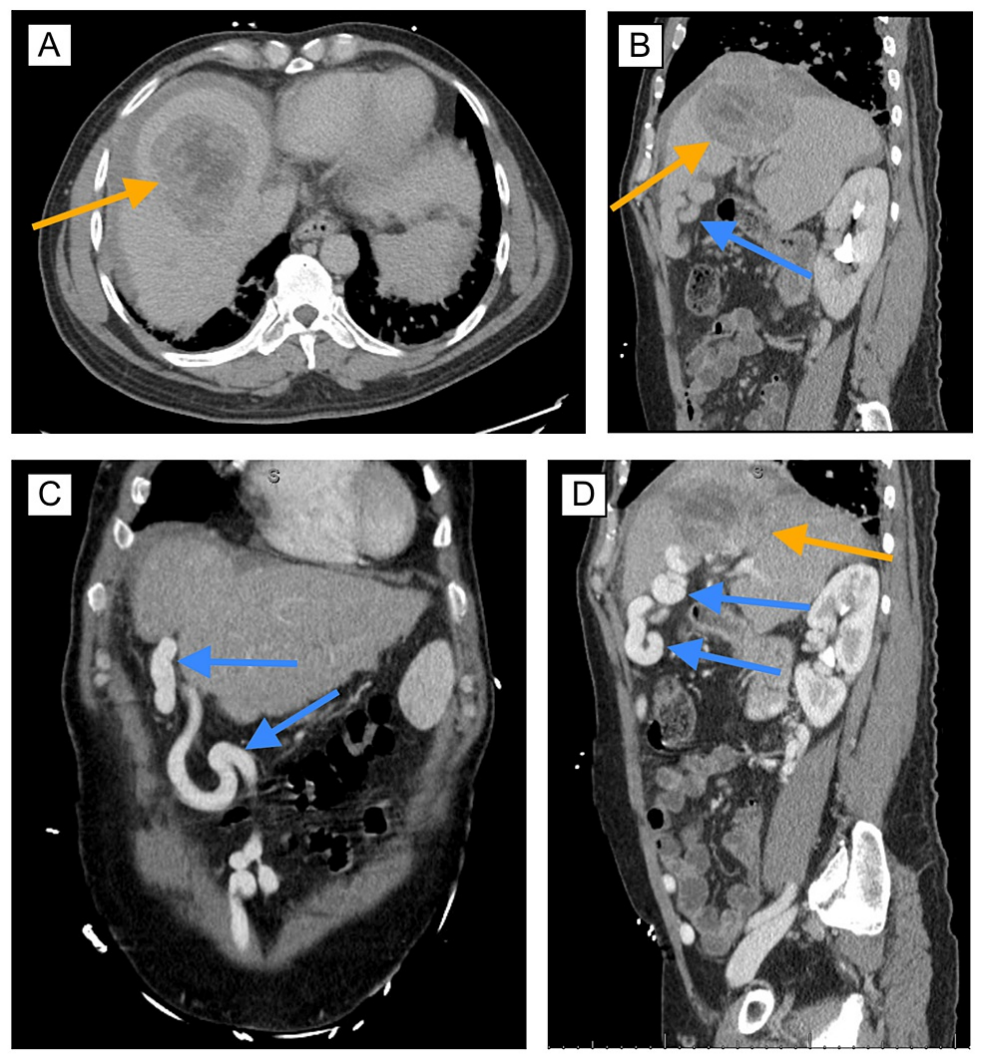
CT imaging of HCC tumor and recanalized umbilical vein. (A/B) CT without contrast showing HCC tumor (orange arrow) and recanalized umbilical vein (blue arrow). (C/D) Venous-phase CT with contrast demonstrating HCC tumor and recanalized umbilical vein. CT: computed tomography; HCC: hepatocellular carcinoma
